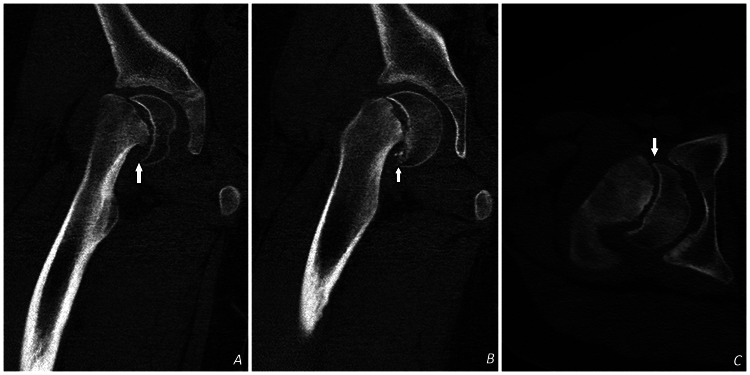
Axial (C) and coronal (A and B) CT scan views of the right hip demonstrating SUFE (white arrows). SUFE: Slipped Upper Femoral Epiphysis
